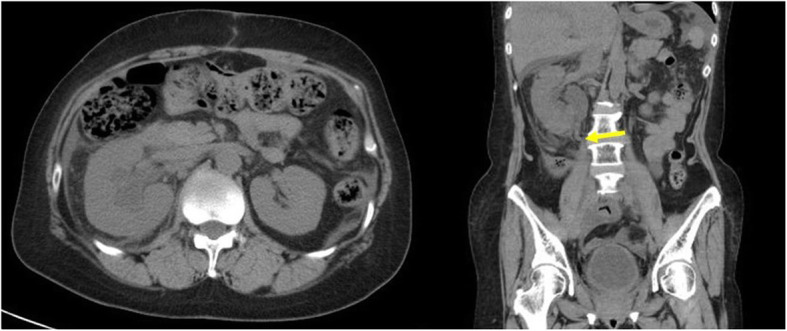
Computed tomography showed a right hydronephrosis in the transverse plane, and the coronal view showed upper ureteral obstruction (arrow)
